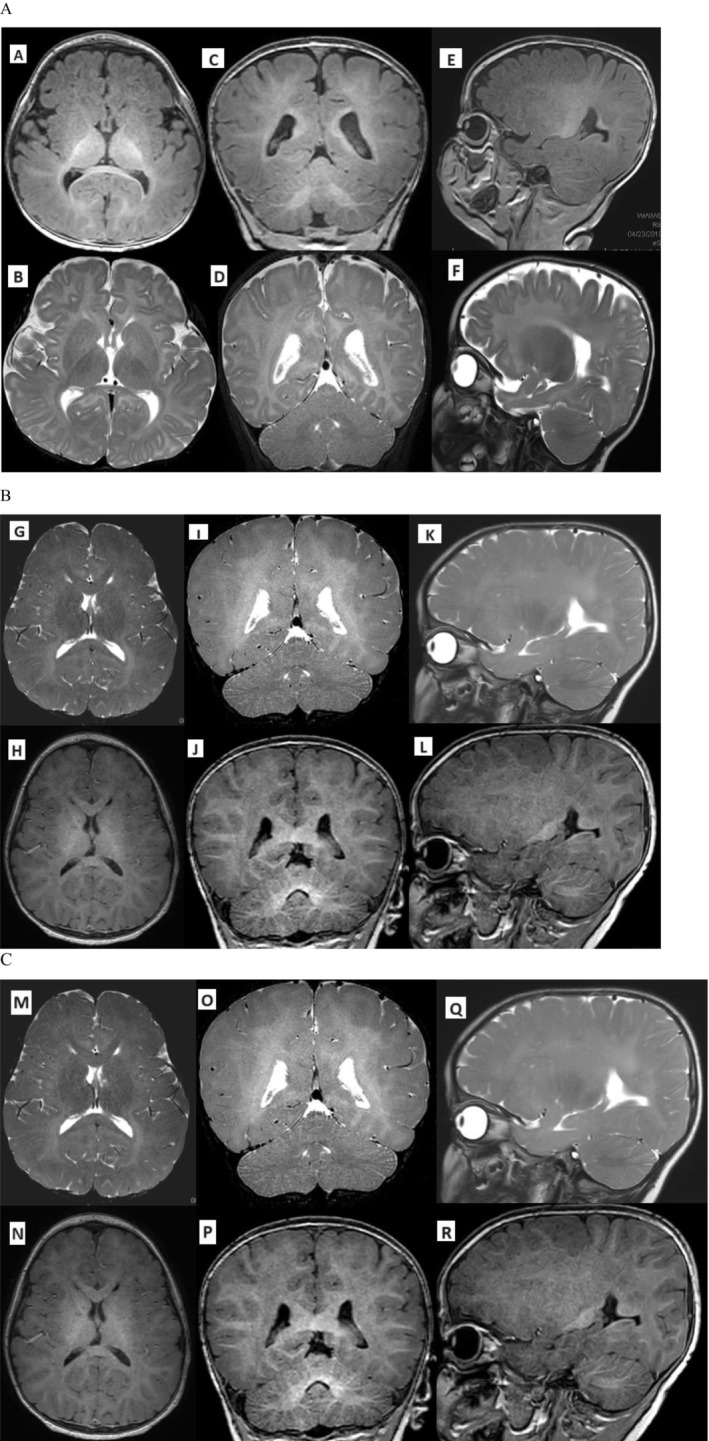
Progressive hypomyelination over serial brain imaging spanning over 4.5 years. (A) Brain MRI (8 months) demonstrated unremarkable imaging findings on axial, coronal and sagittal T1‐weighted (A, C, E) and correspondent T2‐weighted sequences (B, D, F). (B) Brain MRI (at 22 months) revealed deep and bilateral subcortical frontal, parietal, and occipital hyperintense signals on T2‐ weighted images and to lesser extent deep periventricular white matter hypomyelination. The axial, coronal and sagittal T2‐ weighted spaces (G, I, K) and correspondent T1‐weighted images (H, J, L) were normal. (C) Brain MRI (4 years, 2 months) showed diffuse abnormal increased signals on T2‐weighted images in the supratentorial white matter and poor gray‐white—differentiation on T1‐weighted images compatible with hypomyelination. There appears to be further interval loss of normal myelination compared to the prior exams at ages 22 and 8 months old including regions of subcortical white matter abnormal signal currently, previously demonstrating a more normal signal. Overall cerebral volume appears normal T2‐weighted images (M, O, Q) and corresponding T1‐weighted images (N, P, R).
